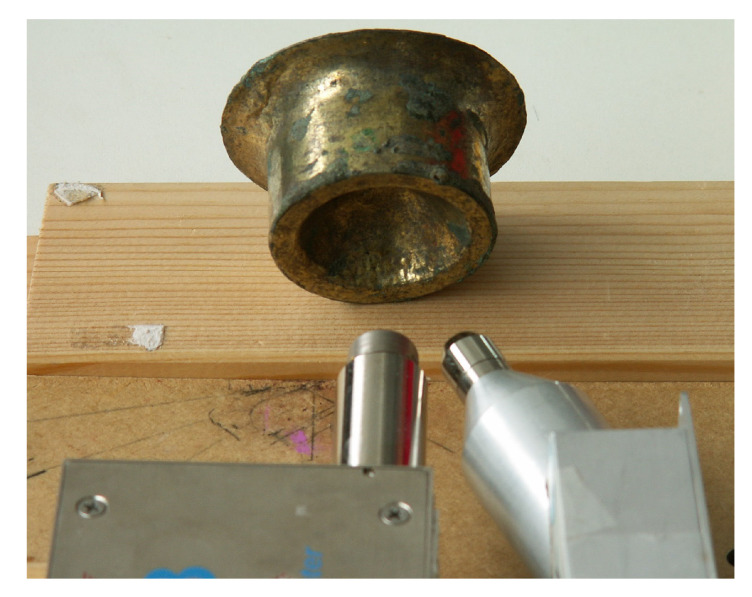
Picture of the experimental setup geometry: Uncollimated detector (on the left) placed vertically with respect to the surface of the sample and X-ray tube usually forming an angle between $30^{\circ }$ and $45^{\circ }$.
